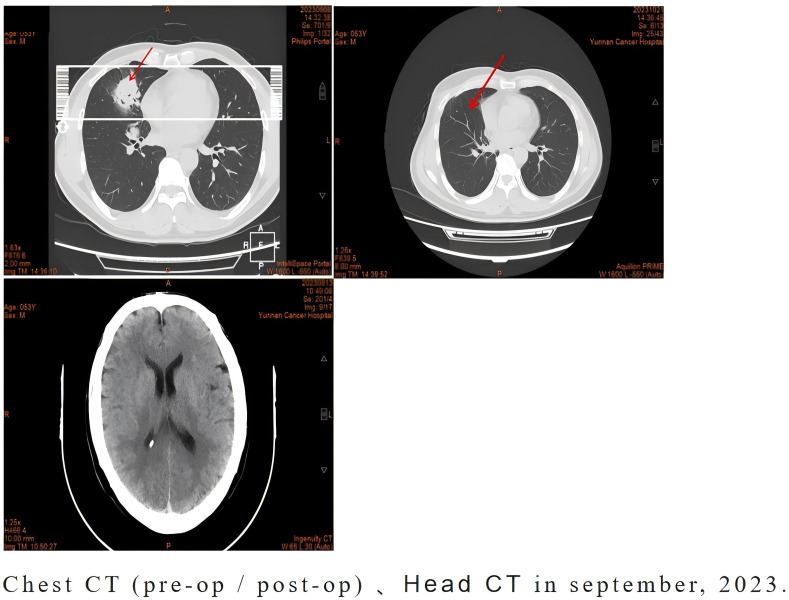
Chest CT (pre-op)/post-op), Head CT in September, 2023.
